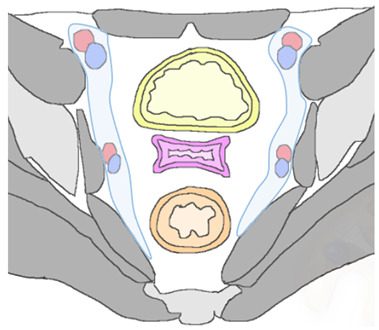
Pelvic sidewall recurrence is defined as a tumor extending within 3 mm and abutting the obturator internus or piriformis muscles with concomitant loss of fat planes. Other findings include: Iliac vessel invasion: vessels surrounded or distorted by tumor (degree of contact > 180°). Ureteral invasions: encased and narrowed ureter by tumor or ureteral obstruction at the level of the tumor. Sciatic nerve infiltration: obliteration of fat planes around the nerves on T1WI, and hyperintense SI at the nerve site on T2WI due to infiltrative edema. Enhancement of peri-neural soft tissue on post-contrast fat-suppressed T1WI is considered a sign of nerve involvement. Bony structures involvement: loss of low SI cortex on both T1 and T2WI, the replacement of the high SI T1W marrow by the intermediate-to-high SI tumor. On post-contrast images, a soft-tissue mass within the bone can be seen. On CT, the pelvic bones may show lytic destructive changes due to direct extension of tumor.
